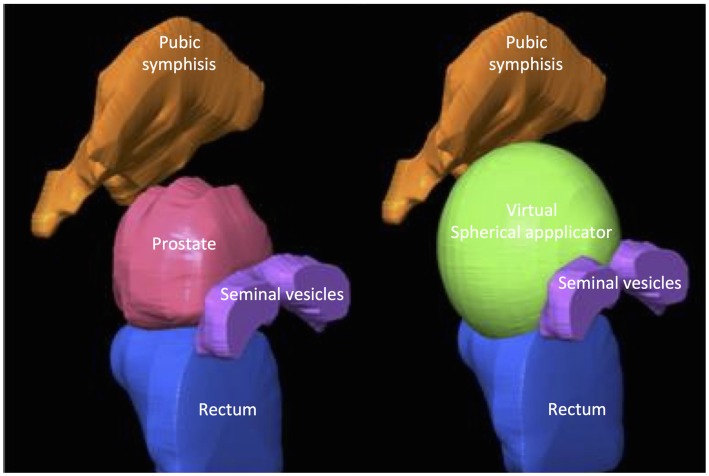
Graphical representation of the determination of applicator size on MRI images.
